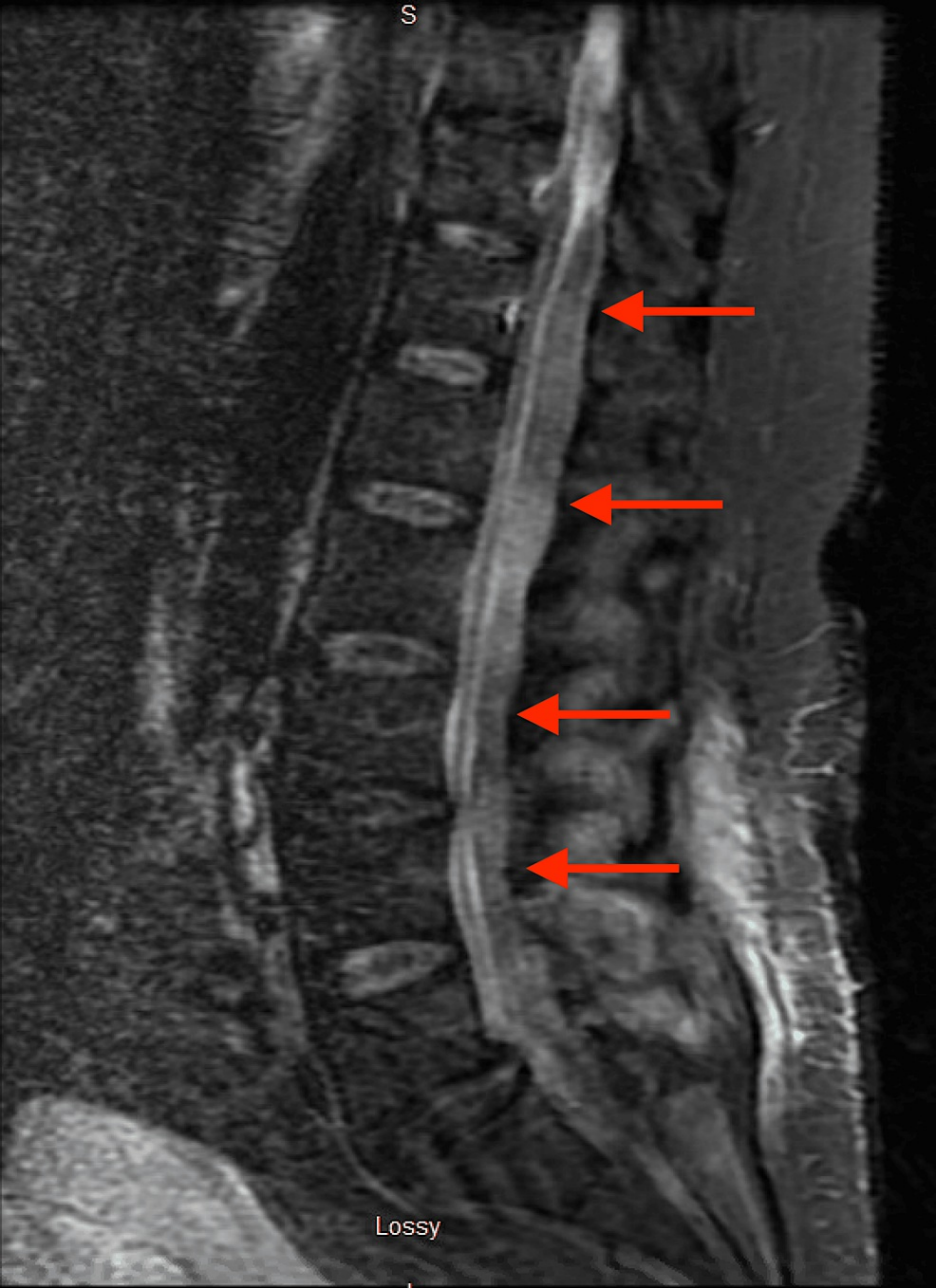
Short-T1 inversion recovery MRI of the lumbar spine without contrast; sagittal view
